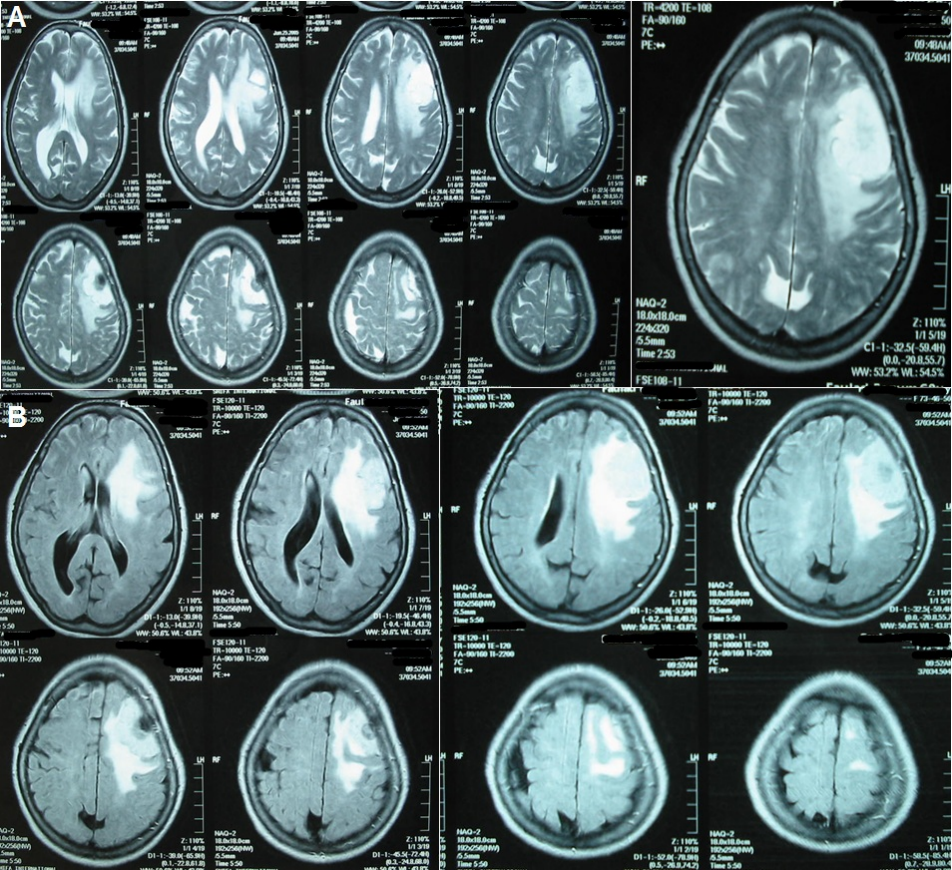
T2-weighted MRI gadolinium enhancement [A]. Axial T2-weighted MRI gadolinium enhancement shows a hyperintense lesion with the central hypointense area. The lesion is surrounded by perifocal edema. [B]. Fluid attenuation inversion recovery (FLAIR) sequence shows a hyperintense lesion with central hypointense area and edema.
